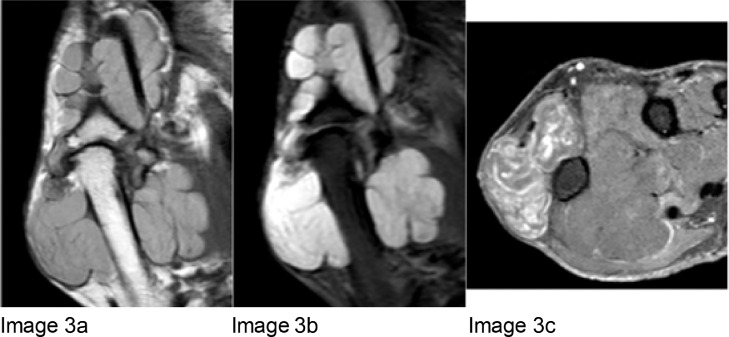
Images 3a-c: Coronal T1 MRI (Image 3a), Coronal T2 MRI (Image 3b), and axial post contrast MRI (Image 3c). The above images display a multilobulated mass encasing the FPL, surrounding the right first proximal phalanx, first metacarpophalangeal joint and first metacarpal, measuring 4.6 × 2.9 × 6.7 cm (width x depth x length). The lesion displays intermediate T1 signal (Image 3a), T2 hyperintense signal (Image 3b) and heterogenous post contrast enhancement (Image 3c).
